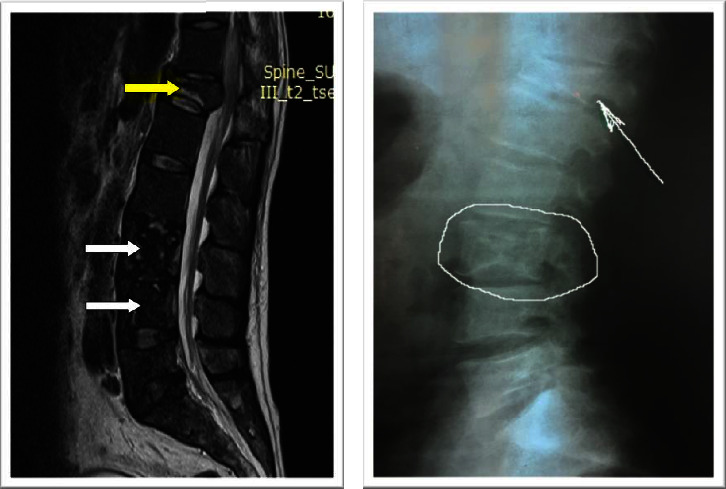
(a) Osteonecrosis of vertebral bodies L3 and L4 (white arrows) and compressive fracture of Th12 (yellow arrow) on MRI. (b) Lateral X-ray of the lumbar spine showing the destruction of Th 12 (arrow) and L3 (circle).
